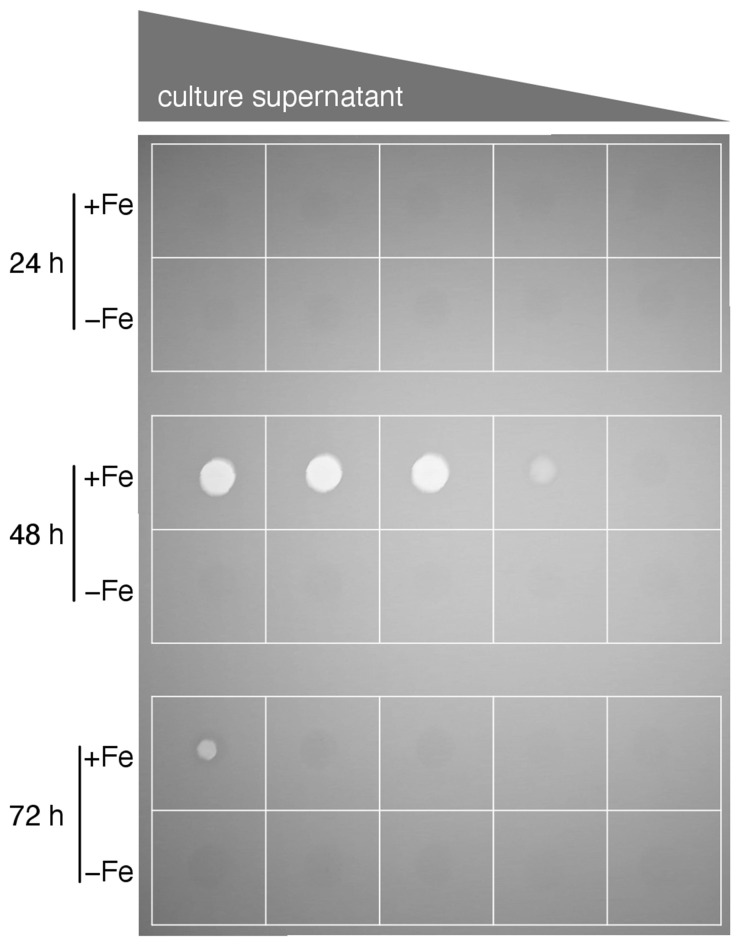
Protease activity is detectable in culture supernatants of +Fe cultures at 48 h and to a lesser extent in 72 h but not in those of younger (24 h) +Fe or in −Fe cultures. Supernatants from cultures were spotted onto an unprocessed X-ray film to test for the presence of proteolytic activities that hydrolyze the gelatin-containing light-sensitive layer (visible as bright circles). Two-fold dilution series (1:1–1:16) in PBS is indicated on top of the picture. The decrease of proteolytic activity in 72 h +Fe compared to 48 h +Fe cultures is most likely caused by degradation of the proteases.
